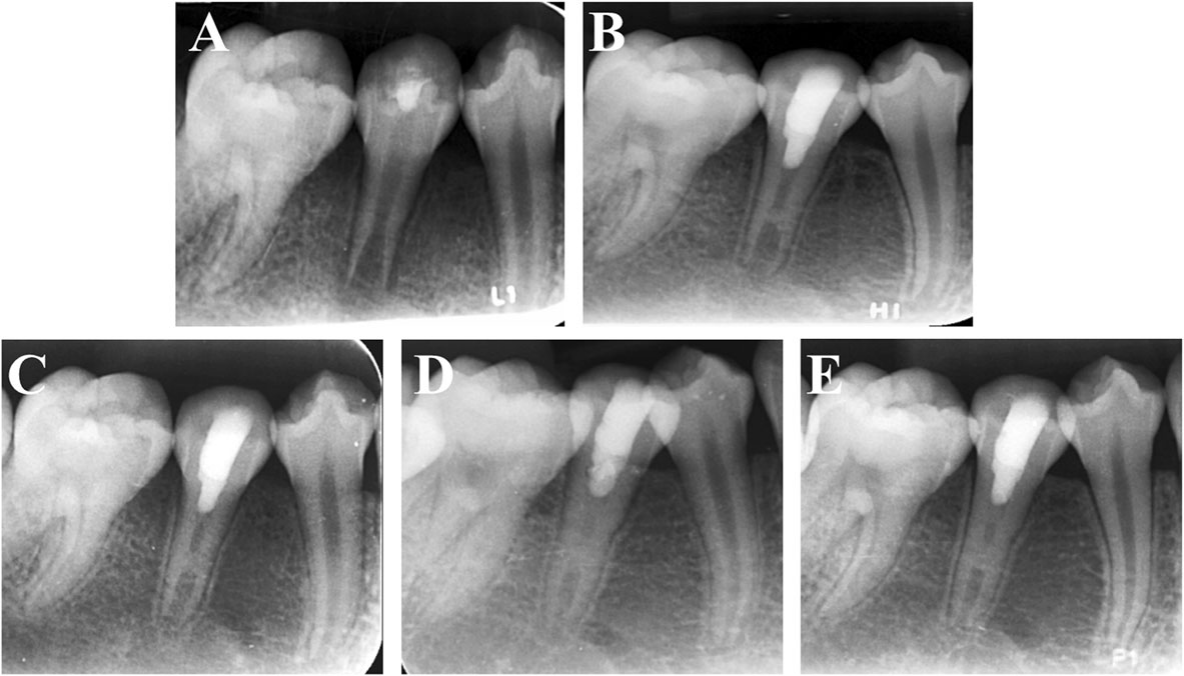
a A periapical radiograph of #45 with apical periodontitis in a 12-year-old girl. In #45, conventional RET was performed. b Three-month follow-up periapical radiograph. c Six-month follow-up radiograph. d Nine-month follow-up radiograph. e Twelve-month follow-up radiograph showing healing of the periapical lesion and root development
